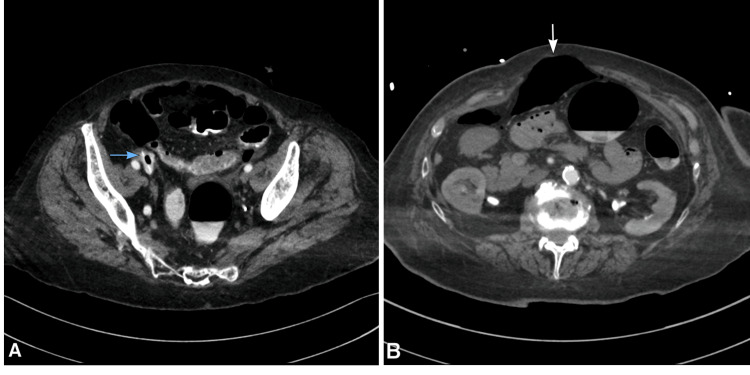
Portal venous phase contrast-enhanced abdominal CT scan, following the colonoscopy procedure The previously described foreign body is situated in the sigmoid colon (A). Extensive extra-visceral gas (white arrow) is observed, suggestive of bowel perforation (B).
